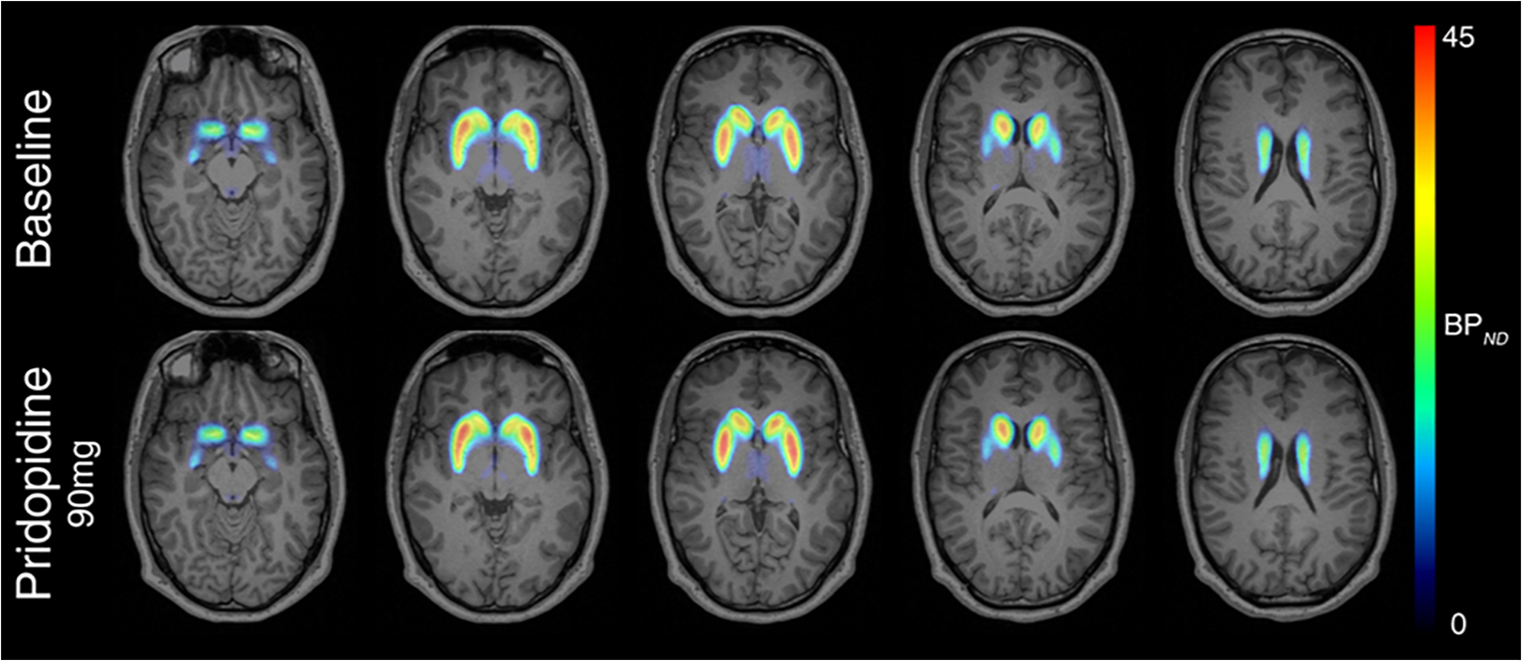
[^18^F] fallypride baseline and post-drug PET of dopamine D2/D3 receptor (D2/D3R) availability in healthy volunteers. Parametric PET/MR images of D2/D3R are demonstrated. There is no D2/D3R occupancy of pridopidine paradigmatically demonstrated in one healthy volunteer as assessed by [^18^F] fallypride PET at baseline and 2 h following single dose of 90 mg pridopidine
